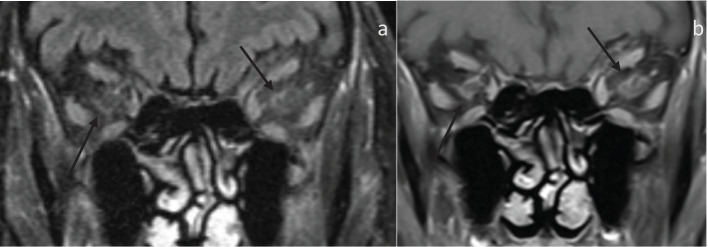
80-year-old presenting with acute left eye vision loss (OS) with jaw claudication, headache, and weight loss. A fundoscopic exam demonstrated left optic disc edema. Subsequent biopsy confirmed GCA. (A) Coronal fat-saturated FLAIR demonstrates FLAIR hyperintensity along the bilateral intraorbital optic nerves sheath (arrows), suggestive of optic perineuritis. (B) The coronal fat-saturated post-contrast sequence demonstrates enhancement of the bilateral intraorbital optic nerve sheath suggestive of perineuritis (arrows).
